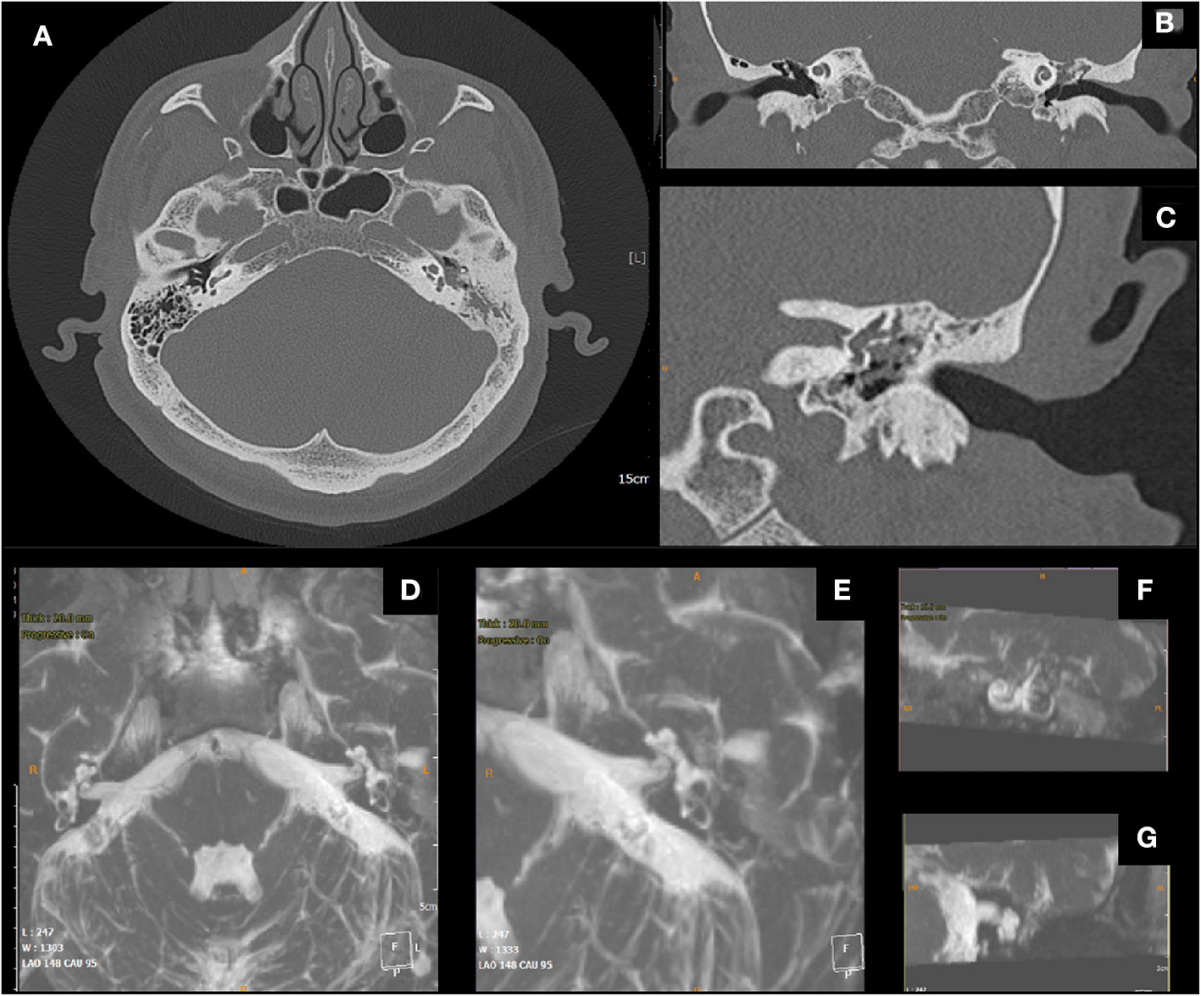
Male, 51 years old; (A) pre-operative axial CT scan, cholesteatomatous tissue eroding the wall of the lateral semicircular canal (LSC); (B) coronal CT scan, erosion of the LSC; (C) detail. (D) Post-operative Axial MRI 3D Fiesta 3D sequences (field of view 20 × 20 cm TR 4 ms; TE 2 ms; flip angle 55°); (E) post-operative MIP for visualization of the cochlea and the semicircular canals, detail of operated side, proper visualization of endocanal fluid showing its preservation (F,G) details.
